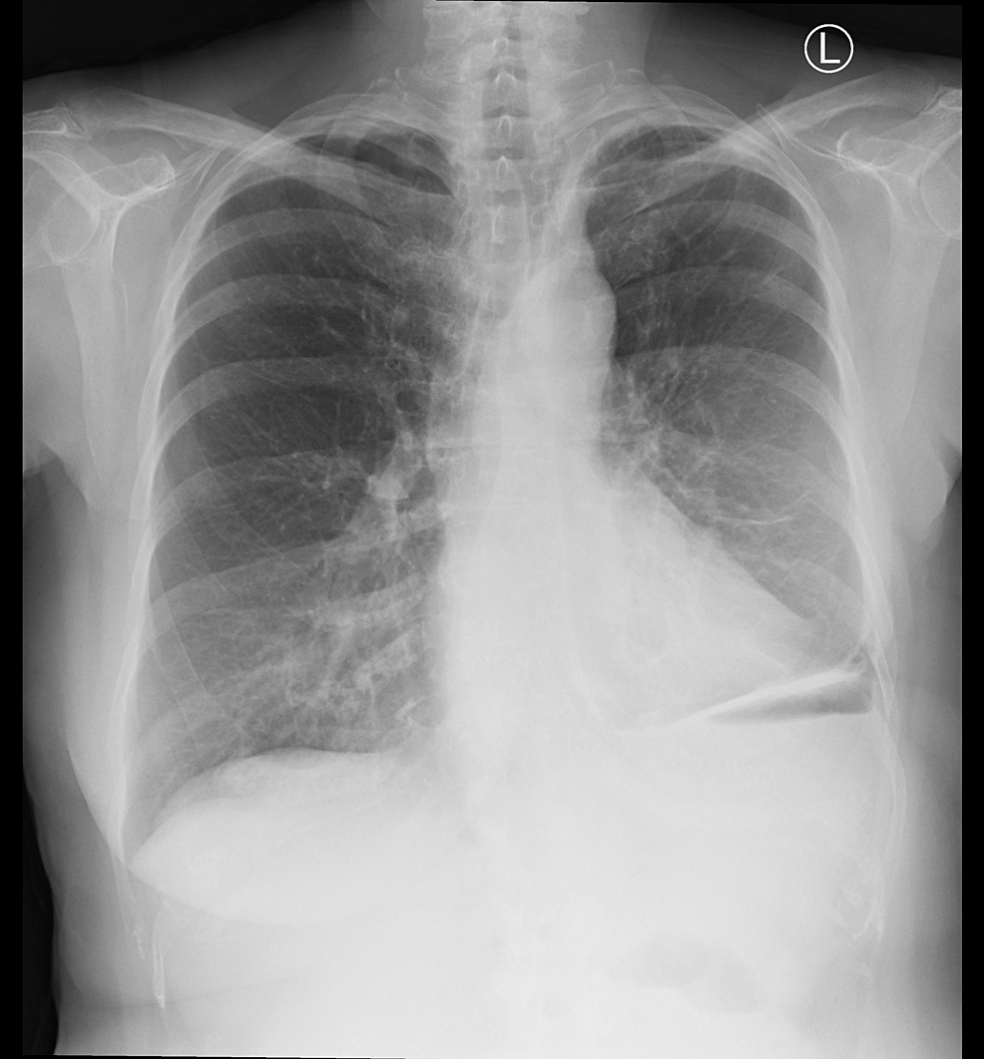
Chest radiograph on admission demonstrating the absence of the stent in the mediastinum.
